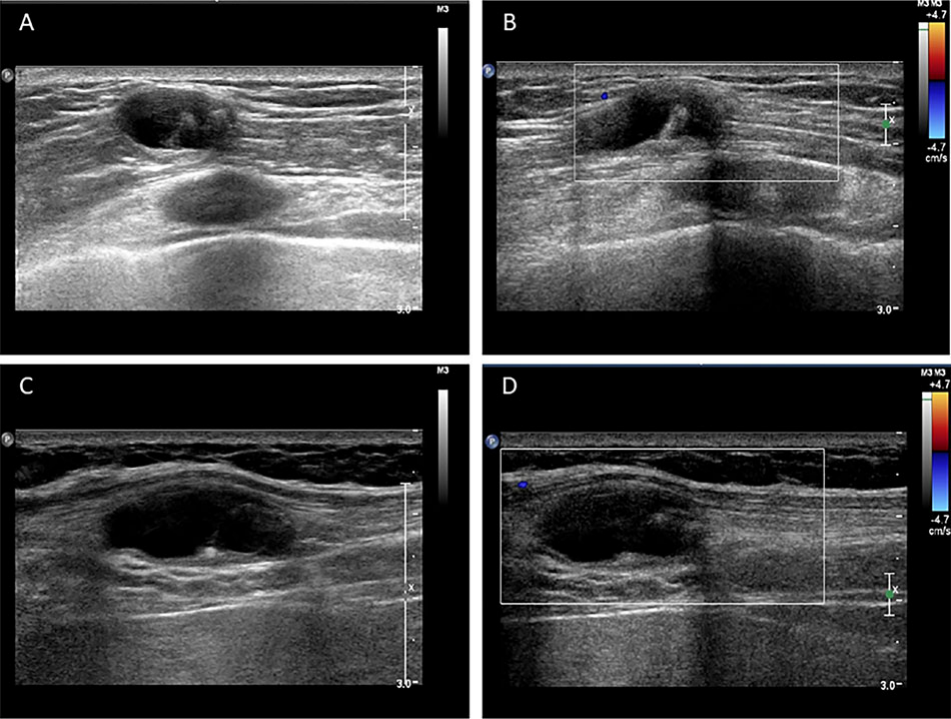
Ultrasound scan shows hypoechoic mass containing cysticercus with calcification in the muscles of (A, B) right anterior thoracic wall (19 × 8 × 15 mm^3^) and (C, D) right midaxillary line (26 × 10 × 19 mm^3^).
